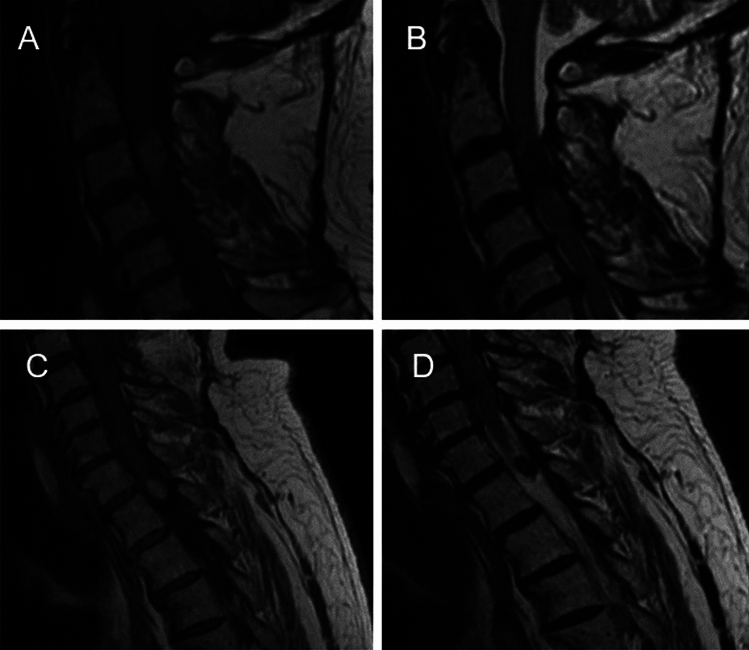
A T1-weighted image of C3 lesion. B T2-weighted image of C3 lesion. C T1-weighted image of T1 (vertebral level) lesion D T2-weighted image of T1 (vertebral level) lesion showing lesions were hyperintense on T1-weighted, and hypointense on T2-weighted MR imaging
